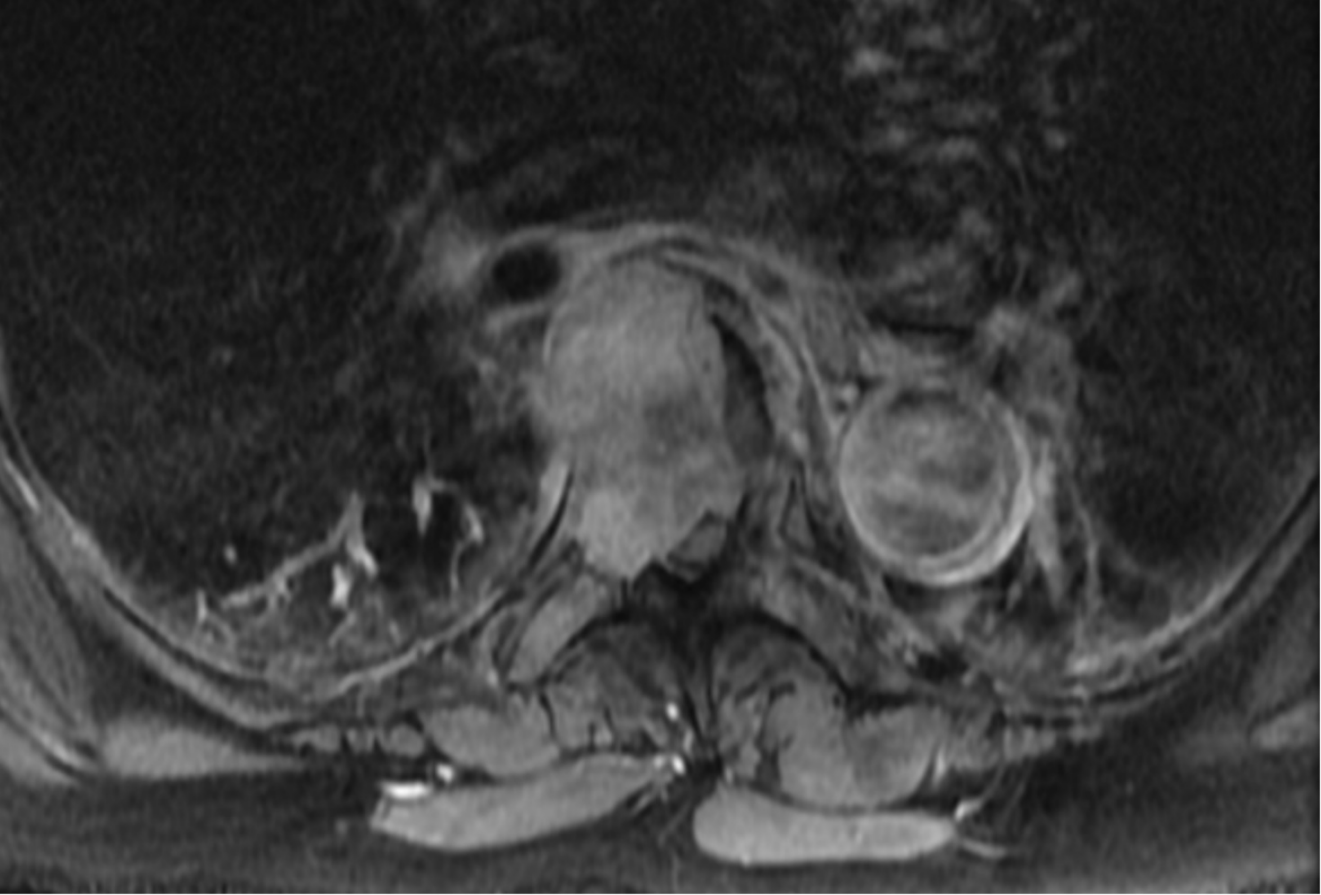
Axial T1 weighted MRI of the thoracic spine with contrast Axial T1 weighted magnetic resonance imaging (MRI) of the thoracic spine with contrast at the level of T7 vertebral body shows the mass extending into the right pedicle, invading into the spinal canal, and displacing the spinal cord to the left.
